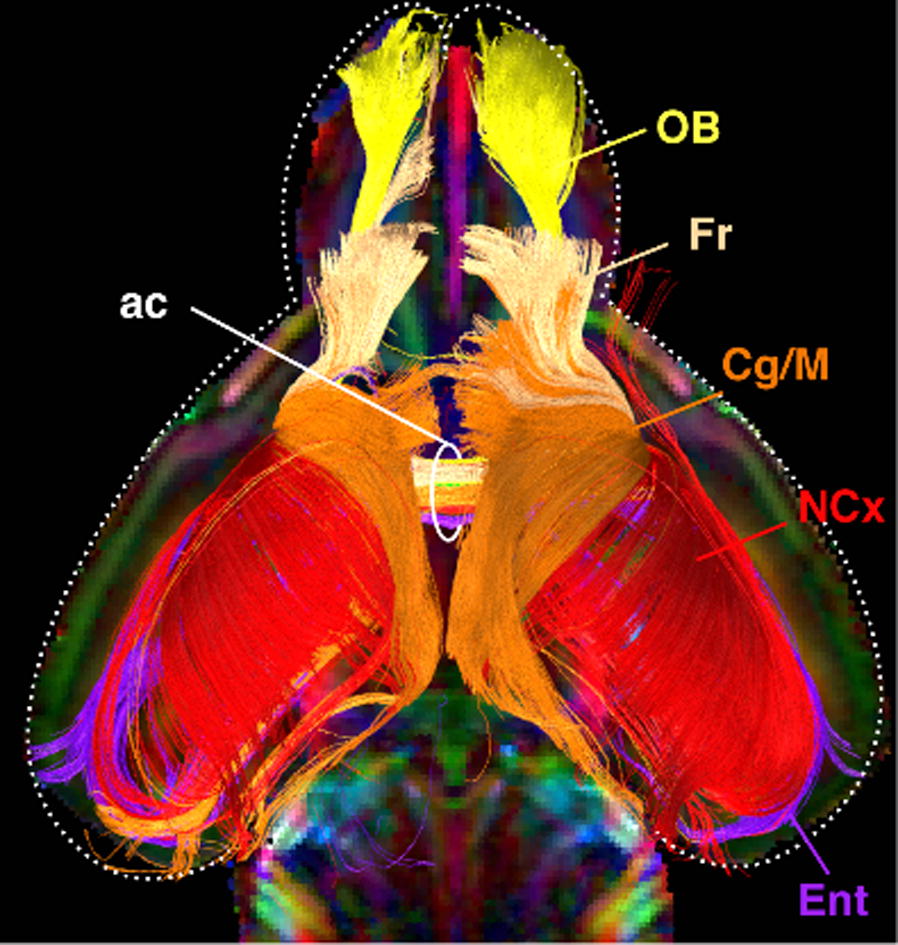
Dorsal view of a fat-tailed dunnart brain, after magnetic resonance imaging and tractography reconstruction. Tracts were generated by parcellating the midsagittal anterior commissure (ac) into five segregated color-coded domains, each labeling homotopic contralateral connections between the olfactory bulbs (OB), frontal cortex (Fr), neocortex (NCx) and entorhinal cortex (Ent)
